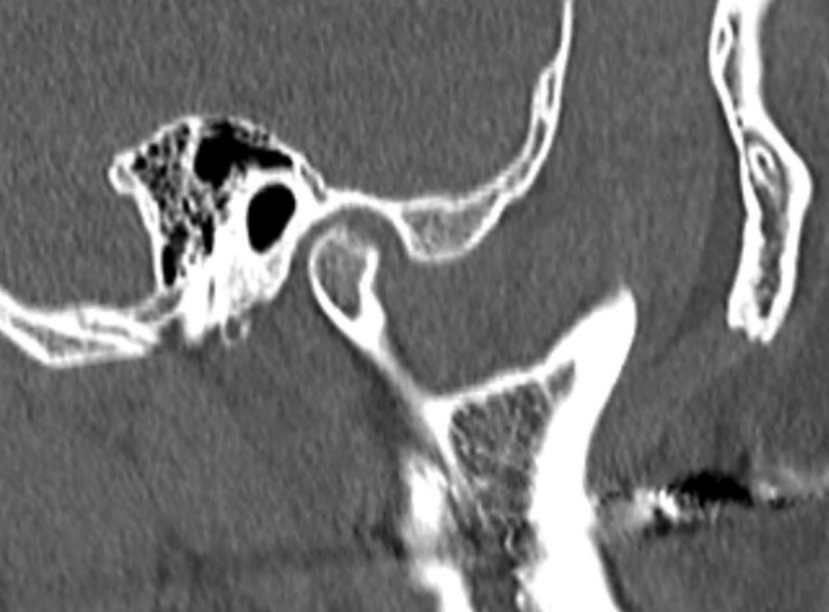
Sagittal cuts of CT which showed erosive osseous changes of TMJ.
